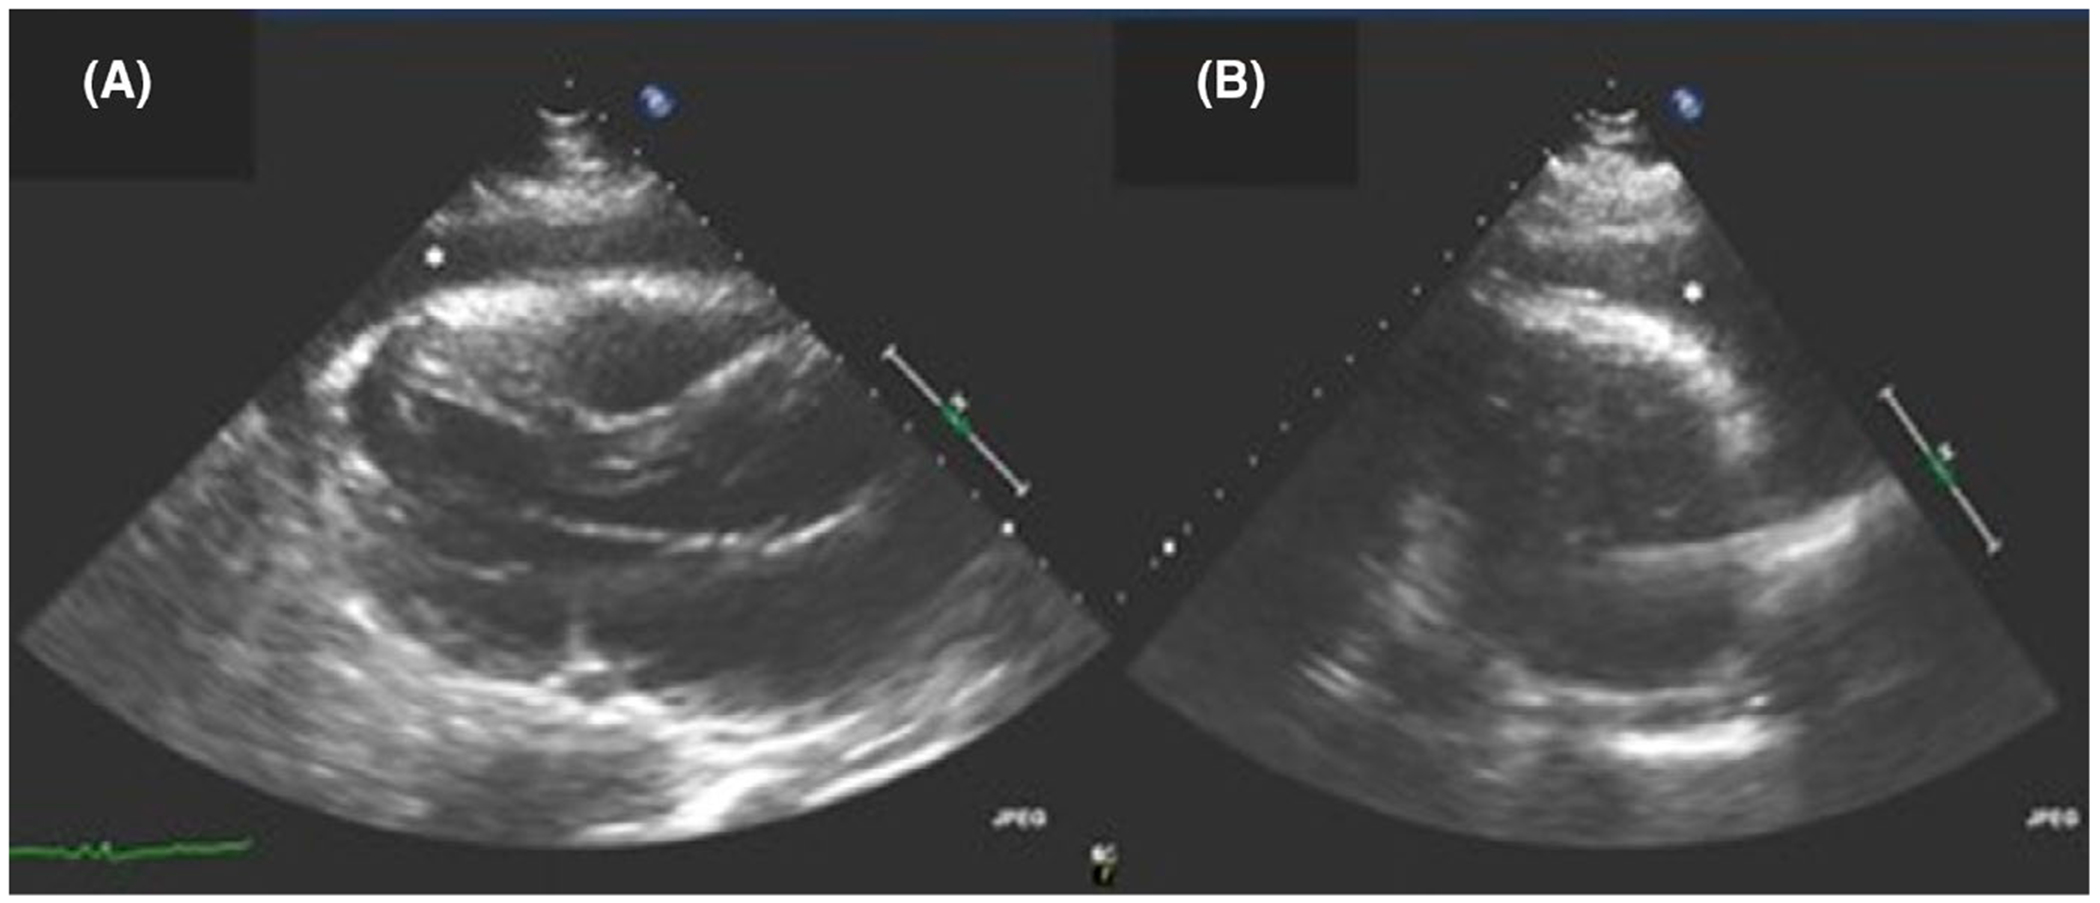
A, An anterior pericardial effusion marked by the * that was seen on the parasternal long axis views on transthoracic echocardiography. B, An anterior pericardial effusion marked by the * that was seen on the parasternal short axis views on transthoracic echocardiography
